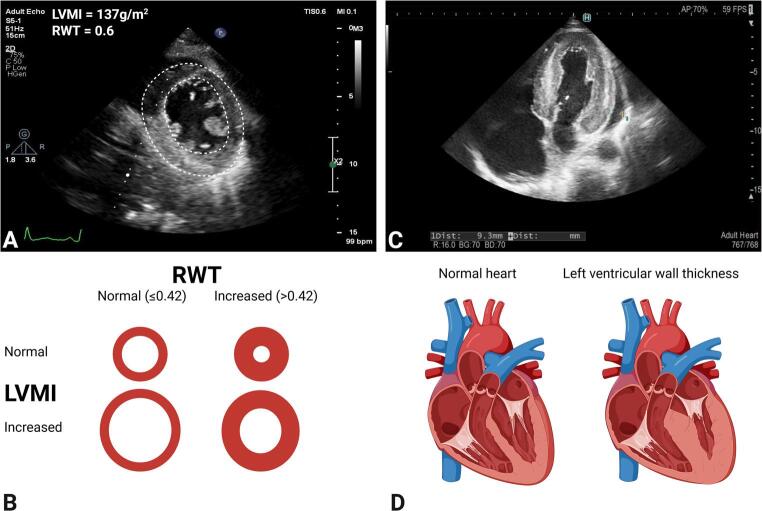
Two-dimensional echocardiographic images of patients with left ventricular hypertrophy. Panel A: Parasternal short-axis view on echocardiography. Panel B: Different phenotypes of left ventricular hypertrophy. Panel C: Apical four-chamber view of a patient with cardiac amyloidosis on echocardiography. Panel D: Anatomical myocardial hypertrophy. Abbreviations: LVMI, left ventricular mass index; RWT, relative wall thickness. Created in BioRender. Dang, N. N. H. (2026) https://BioRender.com/7lclcb1
